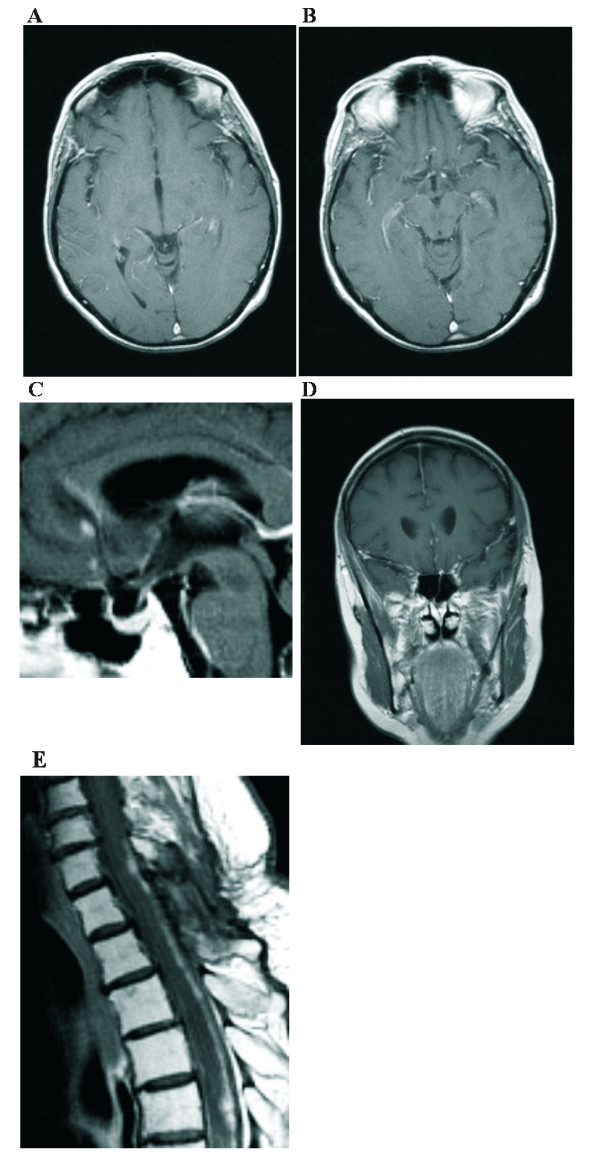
January 2005: Contrast-enhanced axial (A, B), sagittal (C), and coronal (D) T1-weighted MR-images of the brain and sagittal T1-weighted MR-image (E) of the spine demonstrating significant regression of the tumor.
